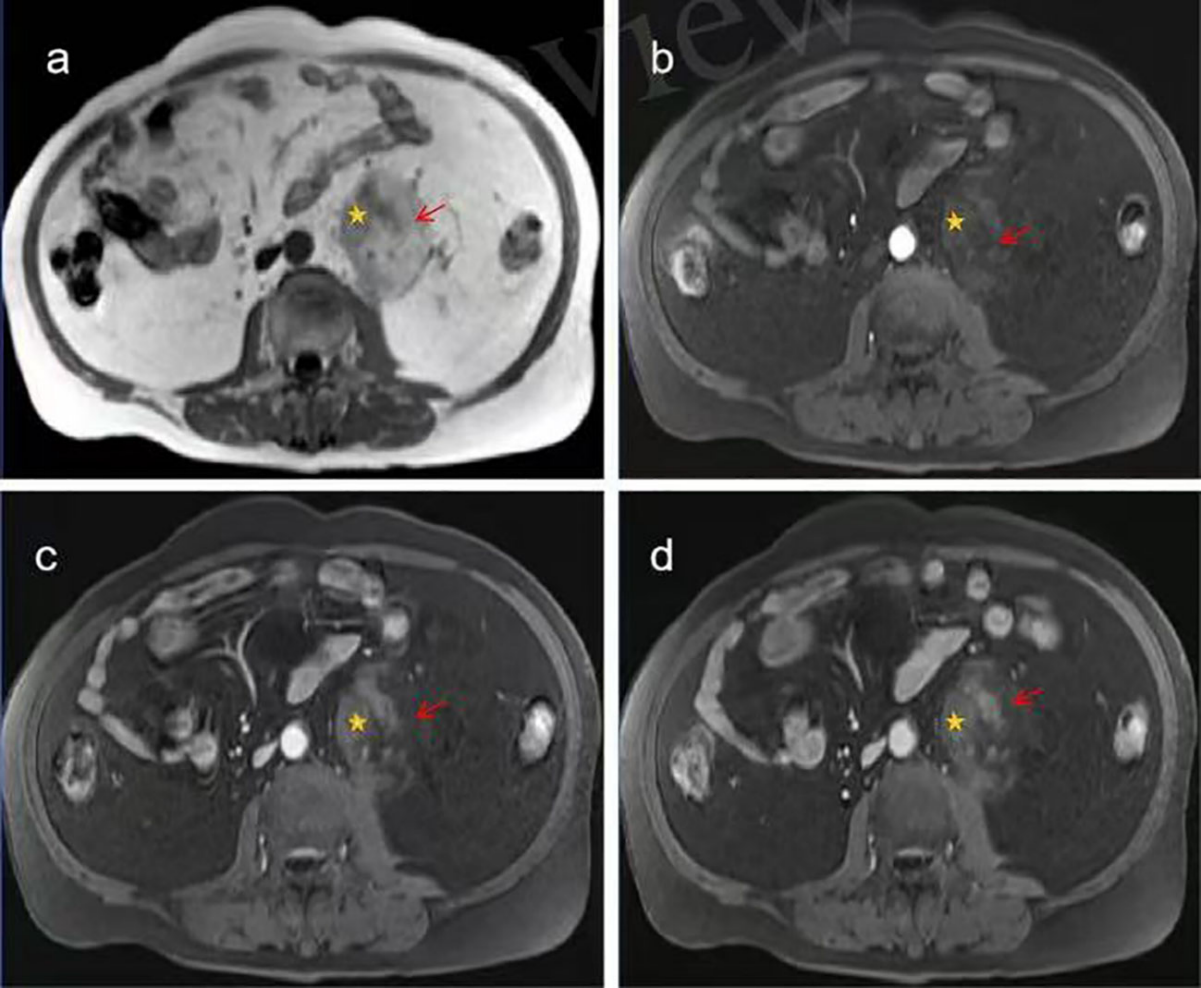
MRI images of extra-adrenal retroperitoneal myelolipoma. (a) Non-contrast abdominal MRI reveals a heterogeneous signal mass containing mature fat adjacent to the left side of the abdominal aorta in the retroperitoneum. (b–d) Contrast-enhanced abdominal MRI demonstrates progressive moderate enhancement in some components of the mass. with no enhancement of the fatty components (Mature adipose tissue, Lipid-containing myeloid tissue).
